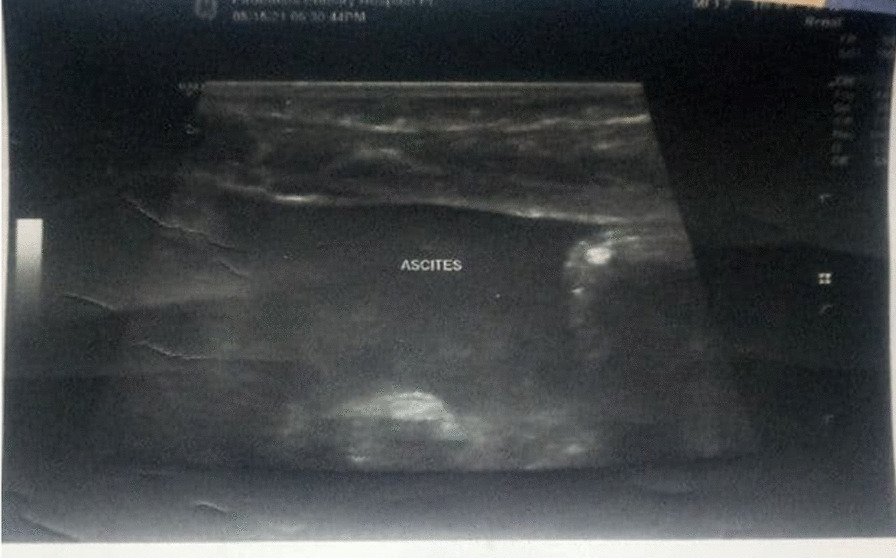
Abdominal ultrasound showing intraperitoneal collection with edematous bowel
